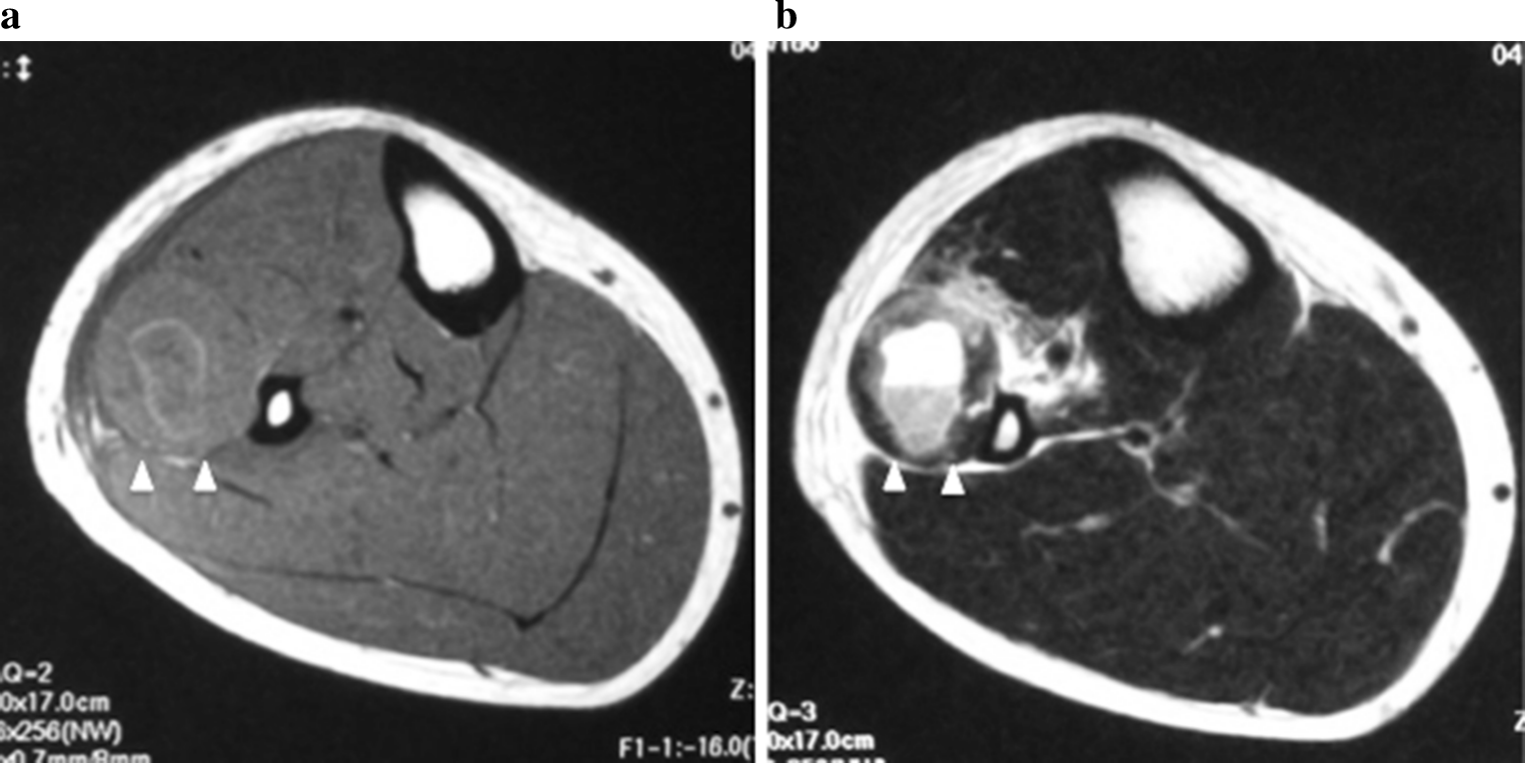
MRI of the right knee (case 1). Axial T1-weighted fast spin echo (a), and T2-weighted fast spin echo (b). *Arrowheads* indicate hematoma in the peroneus longus muscle, which shows a fluid–fluid level
